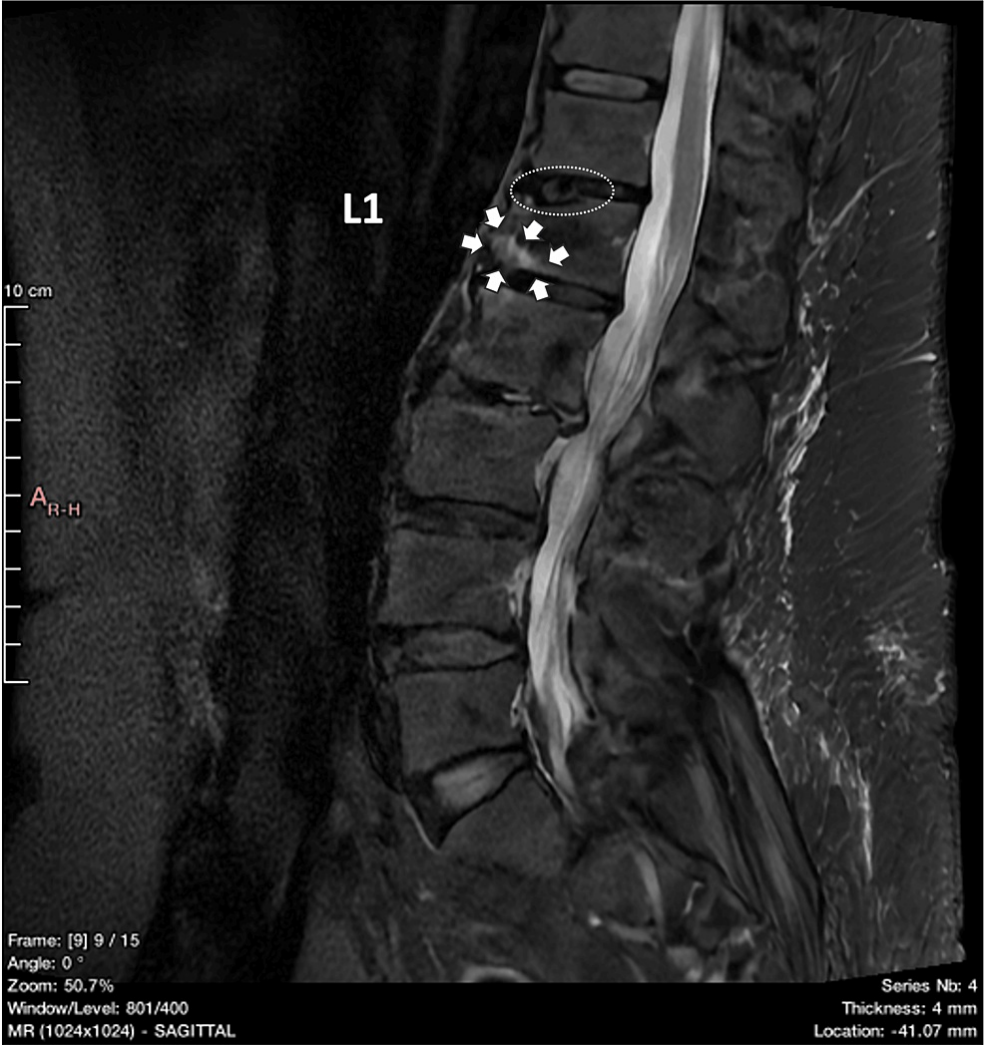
MRI AIR™ Recon DL STIR weighted image (sagittal view) of the lumbar spine obtained at four months following the two balloons kyphoplasty. A Schmorl’s nodule is highlighted by the white dotted oval-shaped line. The small white arrows point at the remaining edema. No gross signs of a malignant tumor at the L1 vertebra, and/or breaching of the vertebral cortex are apparent. AIR™ Recon DL, GE HealthCare Technologies, Inc., Chicago, Illinois, United States STIR: short tau inversion recovery
